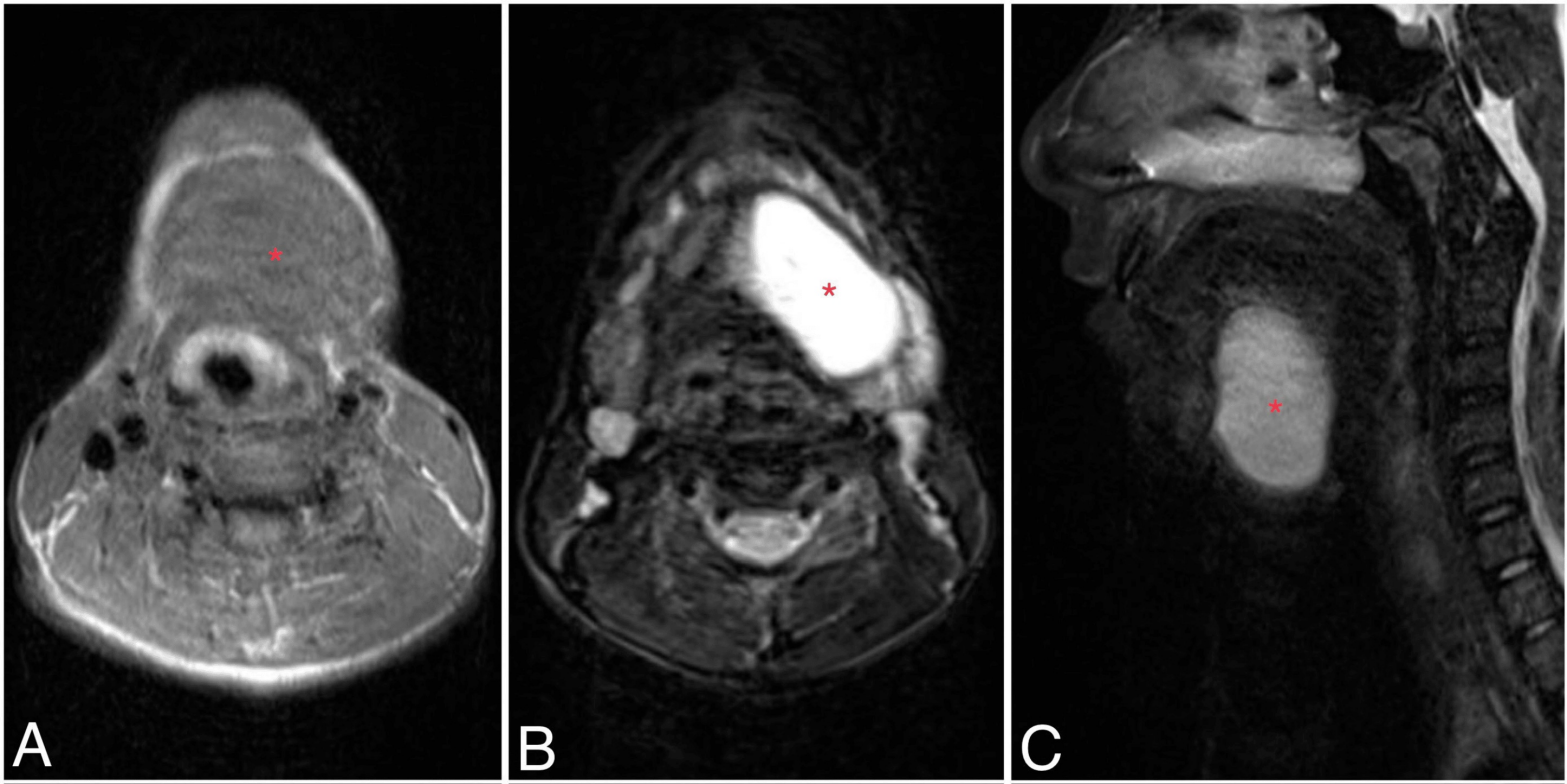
MRI demonstrates a well-defined, thin-walled cystic mass arising from sub-mental space on the left side and extending to the midline measuring 2.5 x 5 x 4.5 in axial, anterior-posterior, and craniocaudal dimensions, respectively. (A) The lesion is characterized by having a homogenous iso-intense signal in the T1-weighted image. (B, C) The lesion is non-enhanced with contrast, and hyper-intense in the T2-weighted image.
